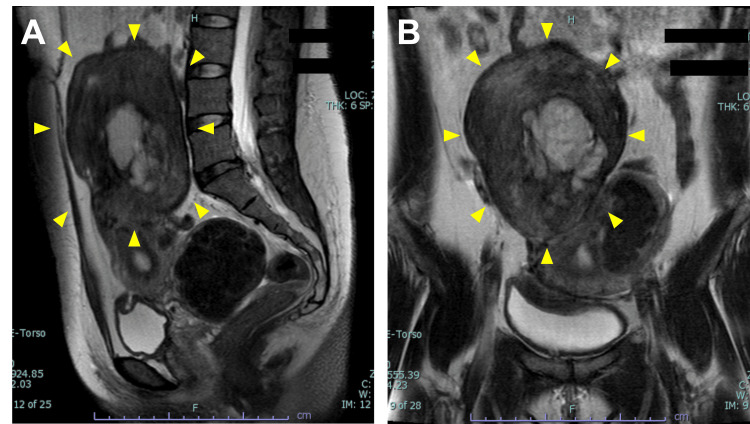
Preoperative magnetic resonance imaging of the pelvis Sagittal (A) and coronal (B) section of magnetic resonance imaging of the pelvis. T2-weighted images showed a 13-cm subserosal tumor (yellow arrowheads) with a hypointense signal. A hyperintense T2 signal was observed in the center of the tumor, suggesting a hemorrhagic component.
